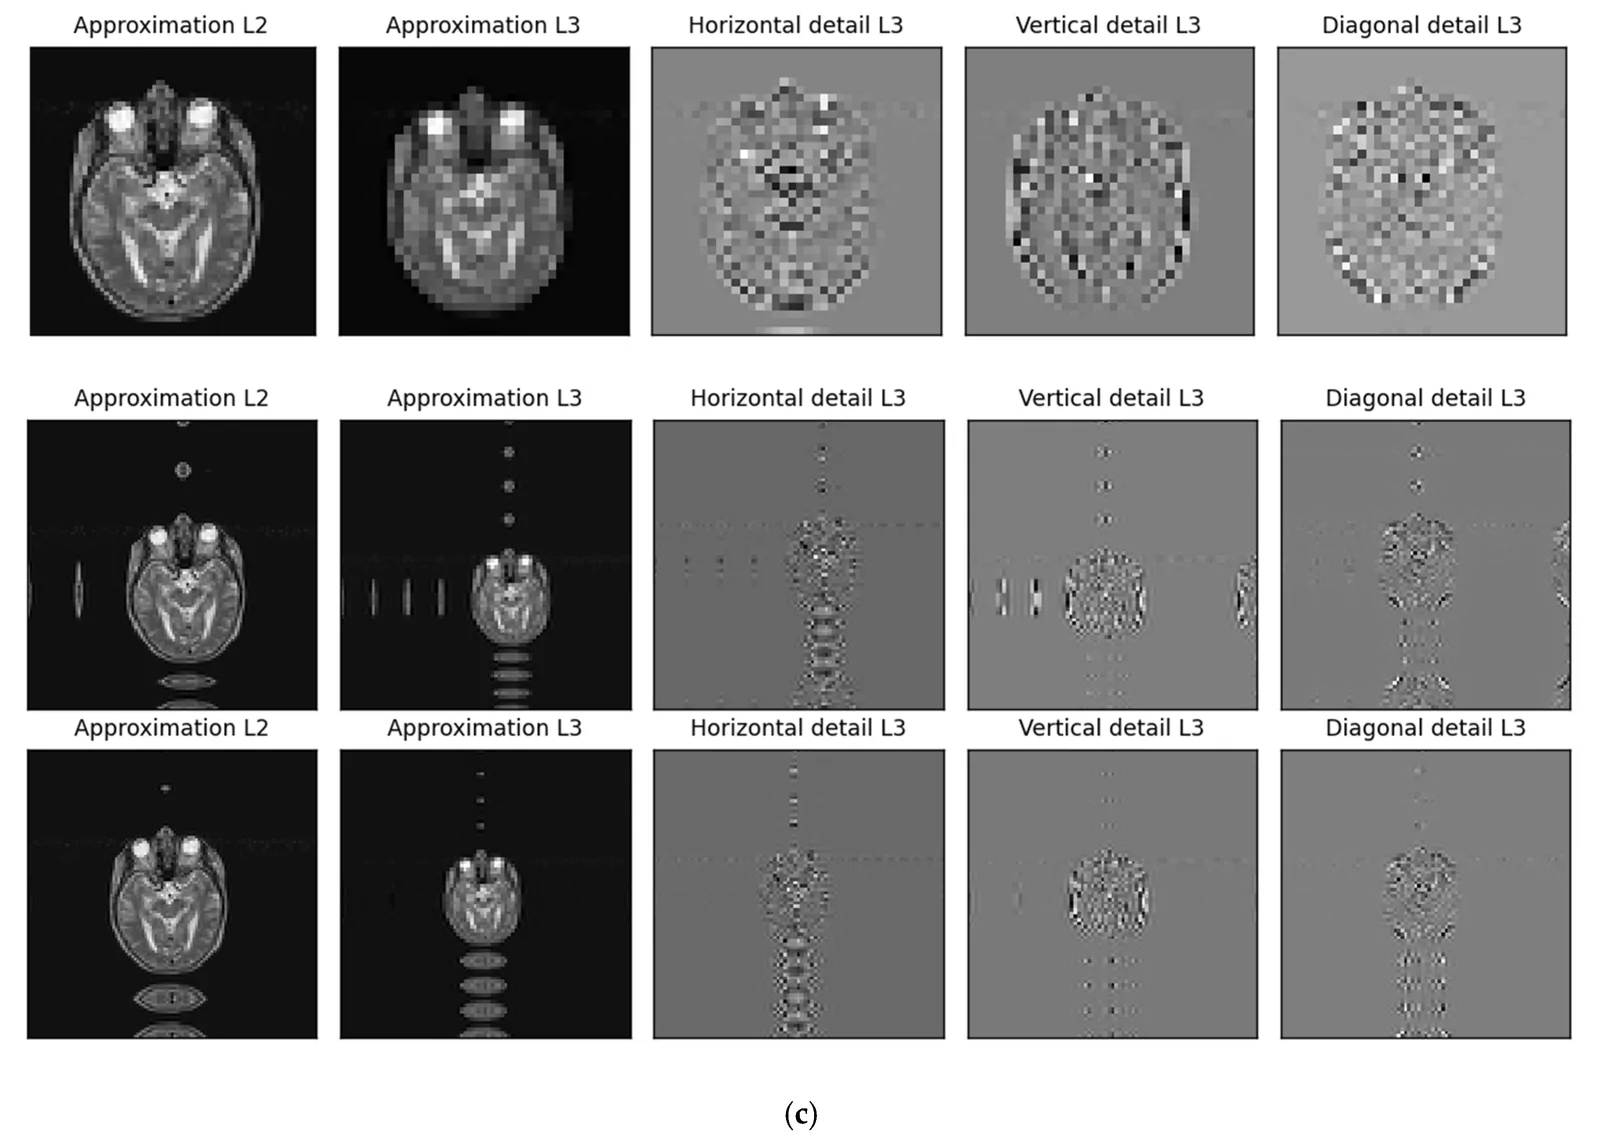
Comparison of the results obtained in the first, second, and third levels of decomposition of an MRI image in Python, using the corresponding wavelets: (a) First level using ‘db1’, ‘db10’, ‘bior1.3’, ‘bior4.4’, ‘coif1’, ‘coif10’, ‘dmey’, respectively; (b) Second level using ‘db1’, ‘db10’, ‘bior1.3’, ‘bior4.4’, ‘coif1’, ‘coif10’, ‘dmey’, respectively; (c) Third level using ‘db1’, ‘db10’, ‘bior1.3’, ‘bior4.4’, ‘coif1’, ‘coif10’, ‘dmey’, respectively.
